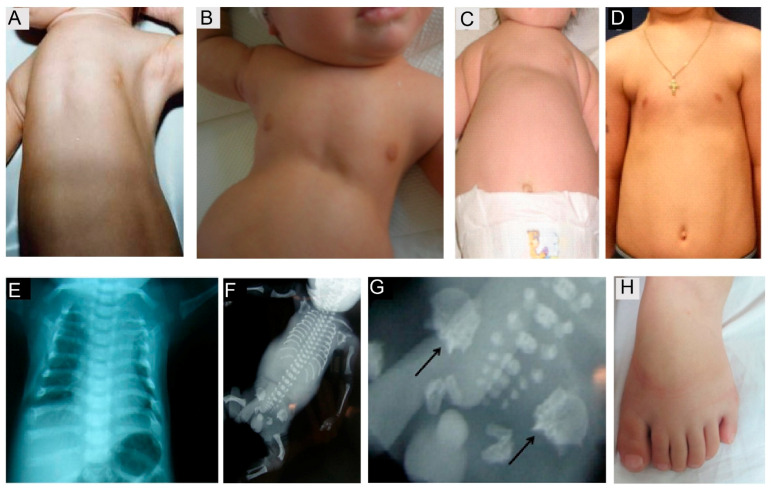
Skeletal features observed in short rib polydactyly syndrome patients. The main hallmark is thoracic narrowing present, observed in utero or from birth (A–C), becoming less pronounced with increasing age (D), (E) short horizontal ribs in a JATD case SRPS case with shortened long bones, long narrow thorax, pelvis configuration with acetabular spurs (F, close up in G, arrows indicate spurs). JATD case with a narrow thorax and shortened ribs, as well as handlebar clavicles, shown in the thorax X-ray, (A) reprinted with permission from Schmidts et al. J Med Genet 2013 May;50(5):309-23 [135], (B) reprinted with permission from Halbritter et al. Am J Hum Genet 2013 *93*, 915–925 [136], (C–E) reprinted with permission from Schmidts et al. Am J Hum Genet. 2013 Nov 7;93(5):932–944 [131]; (F,G) reprinted with permission from Mc Inerney-Leo et al. Am J Hum Genet 2013 Sep 5;93(3):515-23 [133]. Polydactyly (H) is more frequently observed in SRPS compared with JATD, reprinted with permission from Schmidts et al. Nat Commun. 2015 Jun 5;6: 7074 [133].
